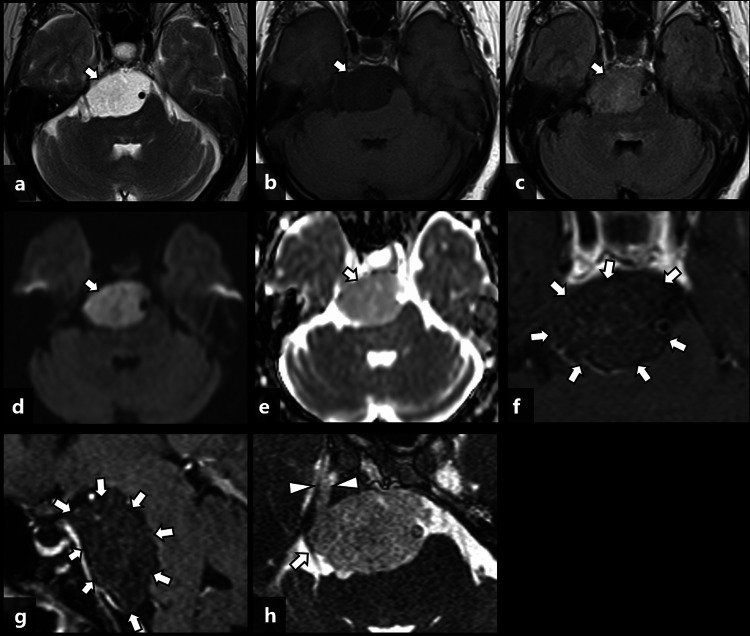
Brain MRI of the patient. The images show (a) axial T2-weighted image (T2WI), (b) axial T1-weighted image (T1WI), (c) axial fluid-attenuated inversion recovery (FLAIR) image, (d) axial diffusion-weighted image (DWI) (b: factor: 1000 s/mm^2^), (e) apparent diffusion coefficient (ADC) map, (f) axial contrast-enhanced T1WI, (g) sagittal contrast-enhanced fat-suppressed T1WI, and (h) axial reformatted image of constructive interference in steady state (CISS). The mass shows markedly high signal intensity on T2WI, similar to cerebrospinal fluid (CSF), and low signal intensity on T1WI (a, b: arrows) but mildly high signal intensity on FLAIR and DWI images (c, d: arrows). The ADC map shows lower signal intensity than CSF and higher signal intensity than brain parenchyma (e: arrow). Contrast-enhanced T1WI shows very faint honeycombed enhancement (f, g: arrows). The tumor extends into the right Meckel’s cave (h: arrowheads), and the right trigeminal nerve is compressed laterally (h: arrow).
